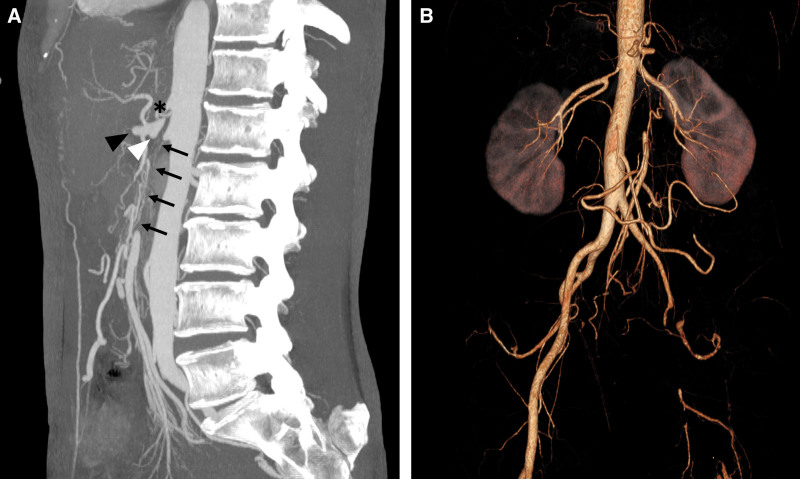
Computed tomography images showing severe stenosis of the celiac artery (asterisk) and thrombotic occlusion of the common hepatic artery (white arrowhead), splenic artery (black arrowhead), and superior mesenteric artery (arrows). (A) Sagittal image. (B) 3-Dimensional reconstruction image.
